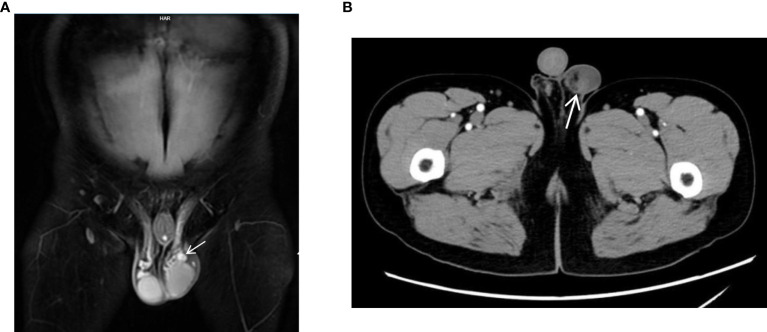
(MRI and CT). (A) Case8, coronal view of enhanced MRI shows a mixed signal nodule (white elongated arrow) in the left testicle sheath and epididymis, significantly enhanced. (B) Case8, enhanced CT cross-section shows a slightly dense nodule (white elongated arrow) above the left testicle which is seen as enhancement.
